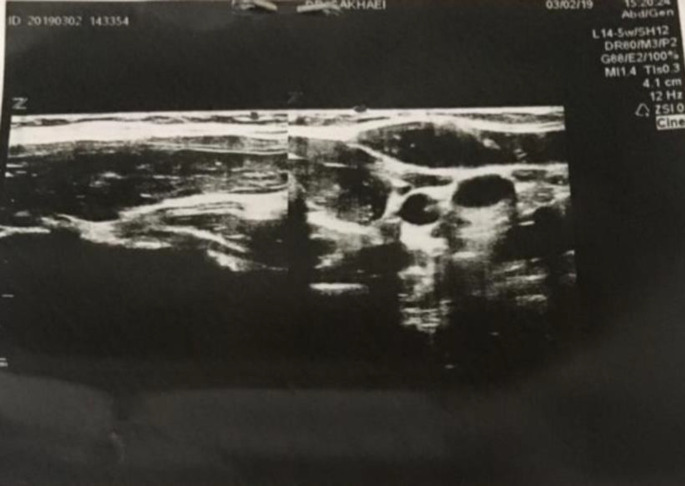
The last thyroid sonography
